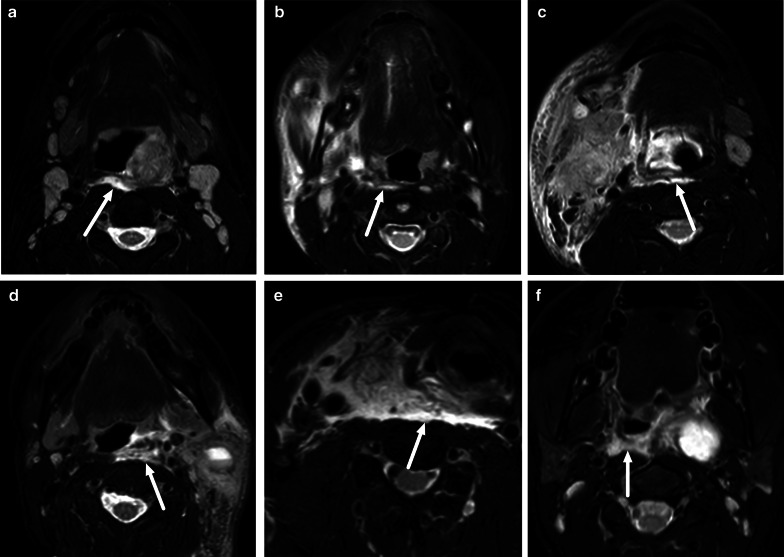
Examples of RPE (arrows) on axial fat-suppressed T2-weighted images in different patients (a–f) with infections of various origins. Image modified from ref. [12]
